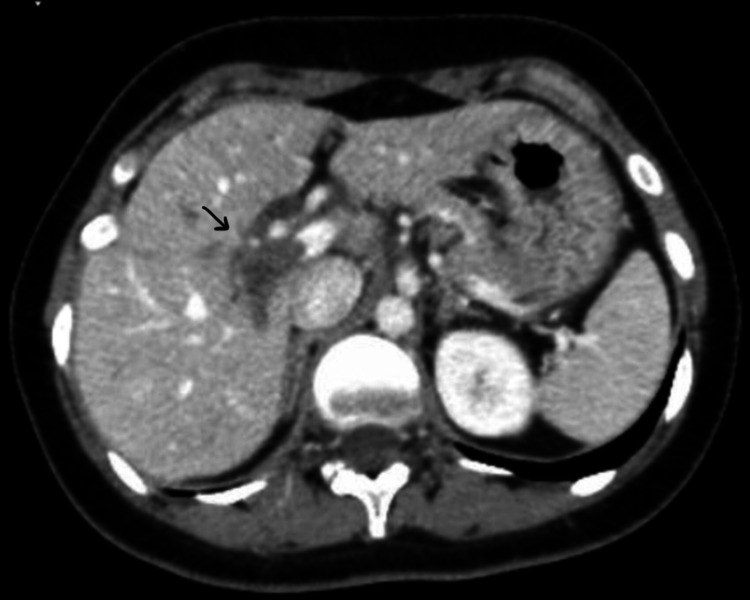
Contrast CT Abdomen (Axial Section)
